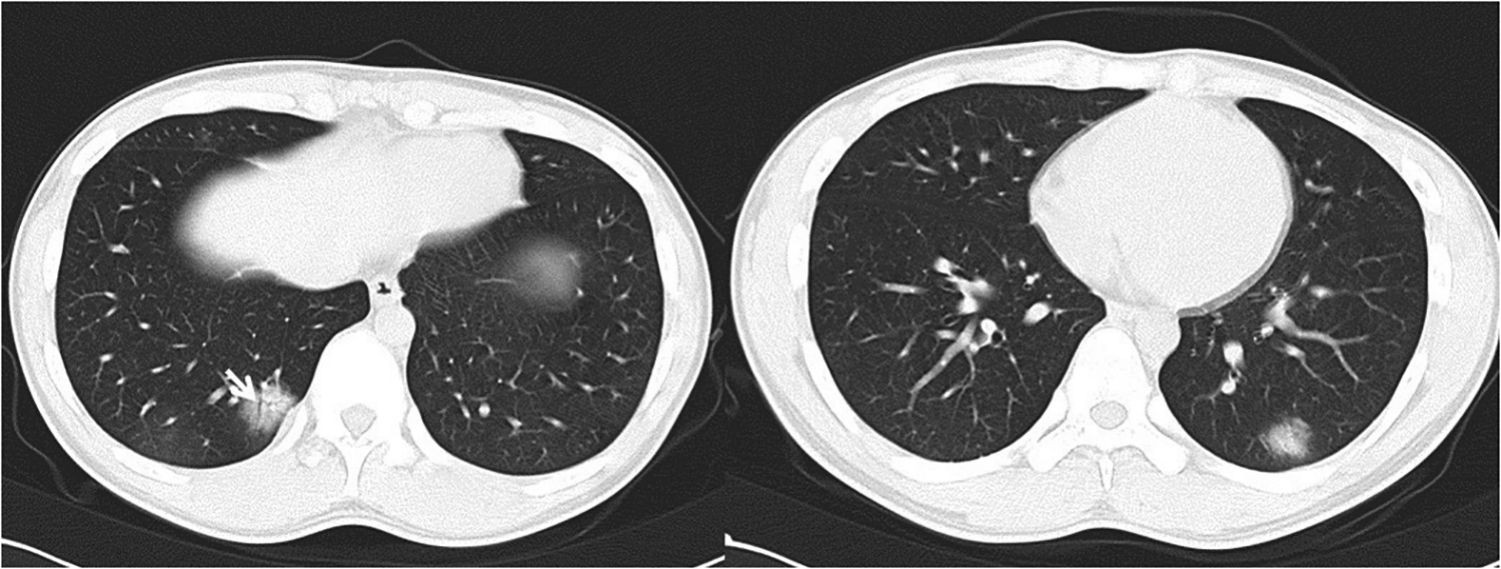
17-year-old boy infected with the original SARS-CoV-2. Initial unenhanced chest CT scans show consolidation with air bronchogram (arrow) in the posterior basal segment of lower right lung and consolidation with ground glass opacities in the posterior basal segment of lower left lobe. These CT findings were assigned CT severity score of 3.
